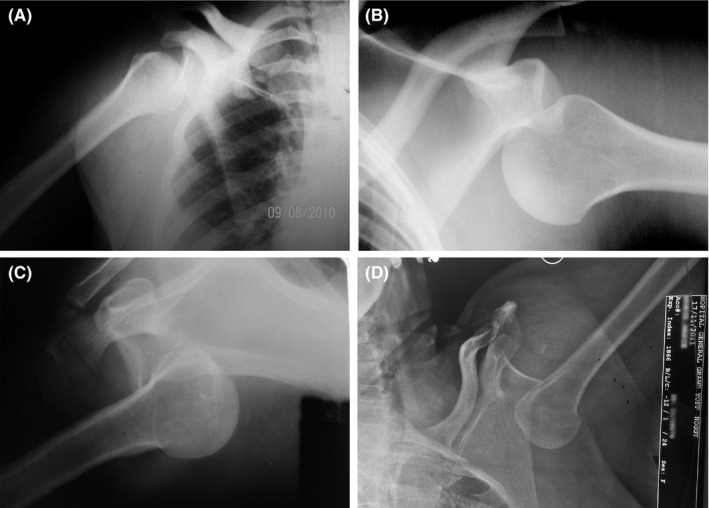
Anteroposterior (AP) view radiographs of shoulders demonstrated shoulder inferior dislocations
